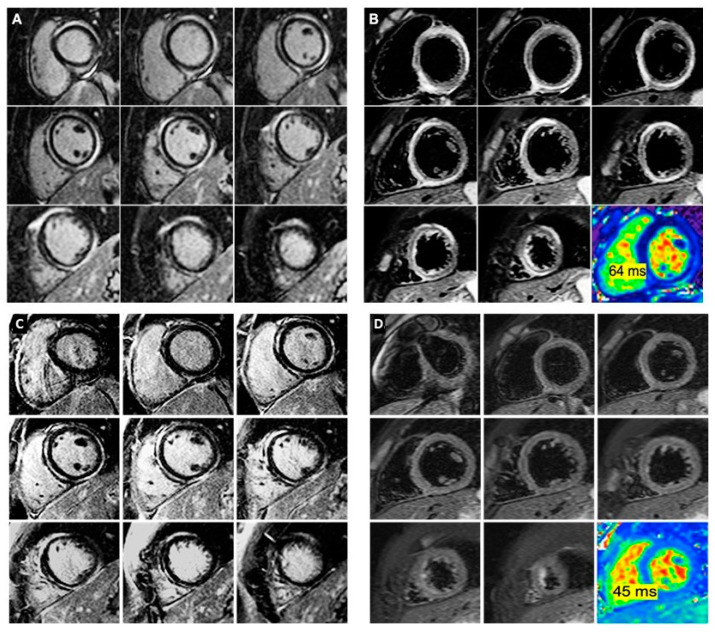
Contrast enhanced cardiac magnetic resonance during the chest pain episode (panels (A,B)) and at the 5-month follow-up (panels (C,D)). (A) Late gadolinium enhancement (LGE) imaging shows circumferential, subepicardial ring-like scarring of the left ventricle walls extending from the base to the apex. (B) Corresponding T2-weighted images showing areas of high T2 signal suggestive of myocardial oedema with similar topography to the scar. The corresponding short axis T2 map is displayed, showing increased T2 time of 64 ms. (C) Late gadolinium enhancement (LGE) imaging shows circumferential, subepicardial ring-like scarring of the left ventricle walls with the same extension as in the initial exam. (D) Corresponding T2-weighted images showing homogenous T2 signal, suggesting the absence of myocardial oedema. The corresponding short axis T2 map is displayed, showing normal T2 time of 45 ms.
